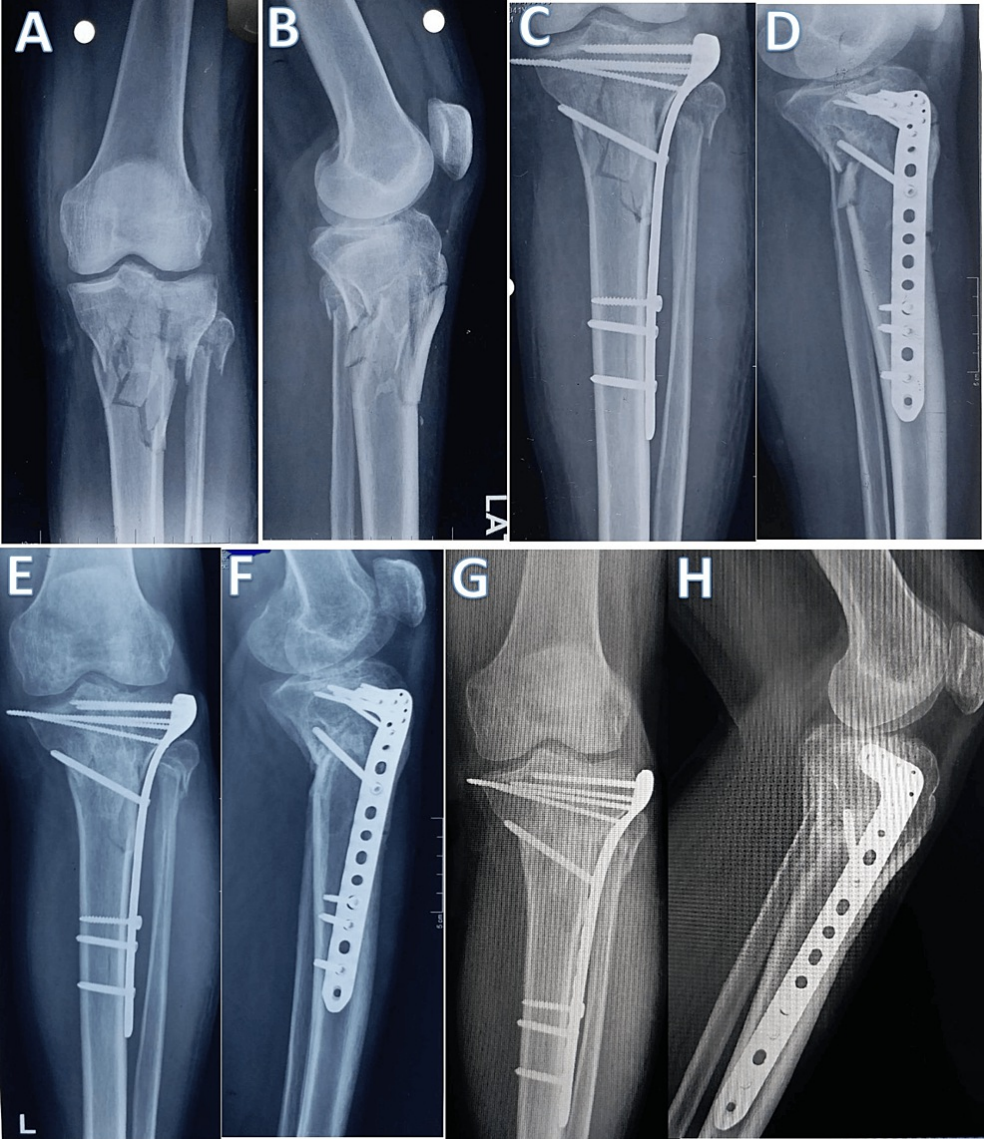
Follow-up radiographs of a 47-year-old male patient with a complex unstable left proximal tibial plateau fracture that was fixed with a lateral locking plate. (A and B) Preoperative radiographs showing the proximal tibial plateau fracture pattern. (C and D) Immediate postoperative radiograph of the fracture fixed by a single plate (lateral locking plate). (E and F) Follow-up radiograph six months after surgery. (G and H) Follow-up radiograph 12 months after surgery showing varus angulation.
